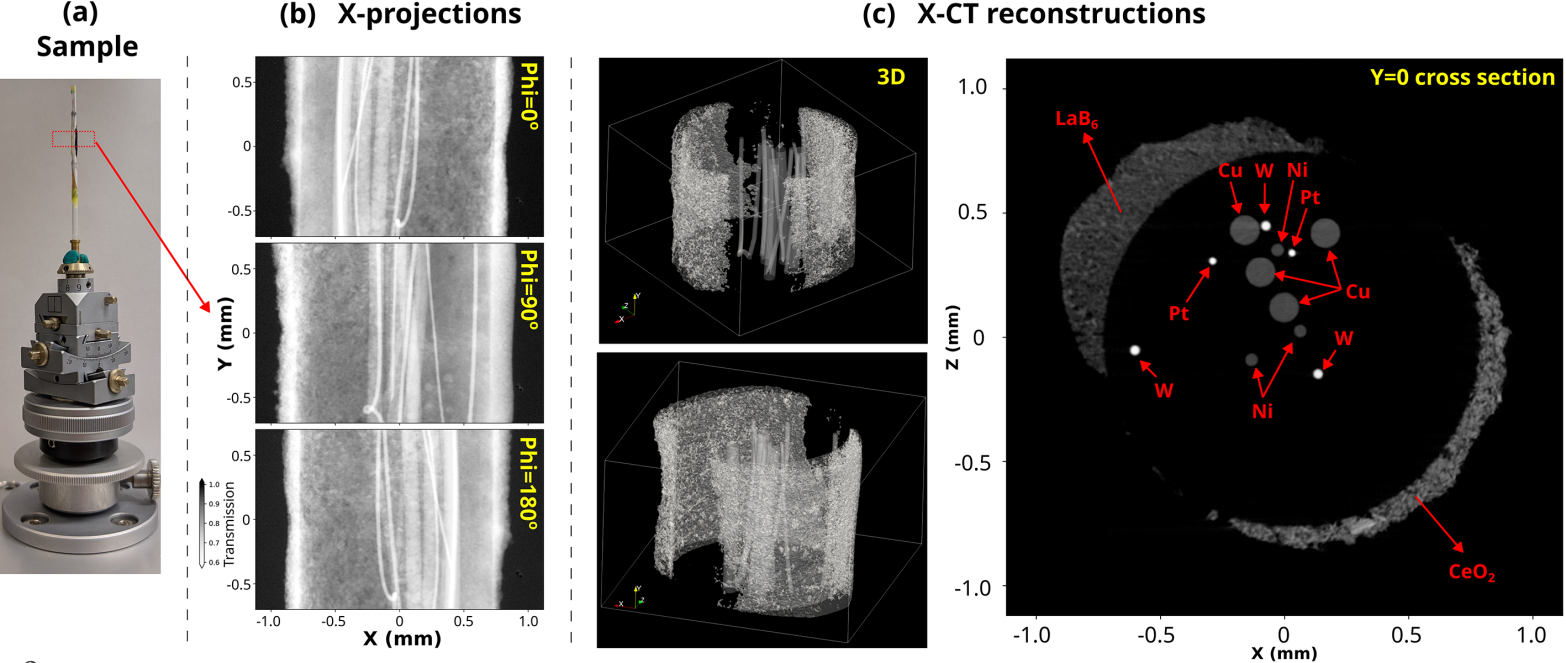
(*a*) Photograph of the demonstration sample used in this study. The dashed red rectangle shows the approximate location of the sample probed by the X-rays. (*b*) X-ray projections (radiographs) of the sample at three different Phi angles. (*c*) Reconstructed 3D X-CT volume of the sample and one *Y* = 0 cross section of the 3D reconstructed volume. The constituents of the sample are highlighted by the arrows. Quartz and Al_2_O_3_ regions cannot be identified in this 2D map.
